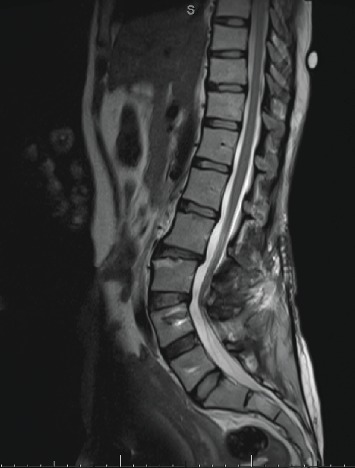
MRI was done 6 months postoperative fixation which revealed normal neurological strutures with no evidence of paraspinal cold abscess formation.
